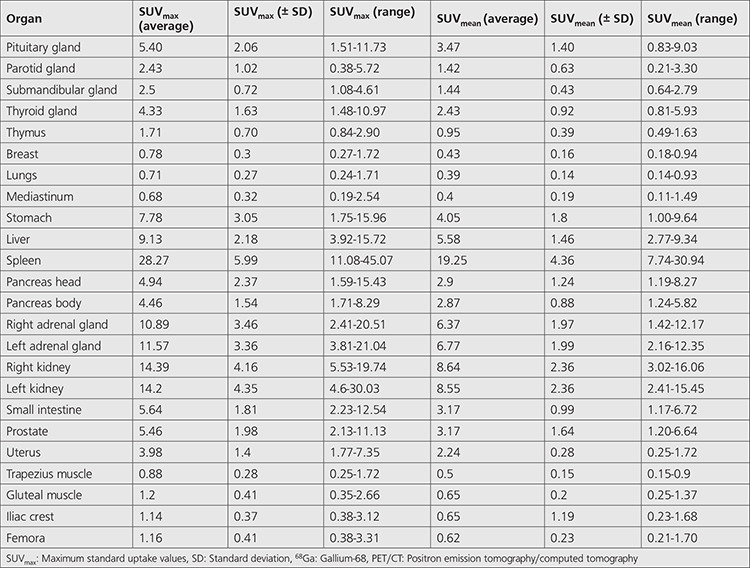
Average SUV_max_ (± SD), average SUV_mean_ (± SD), and range of uptake of ^68^Ga DOTA-TATE PET/CT for all organs
